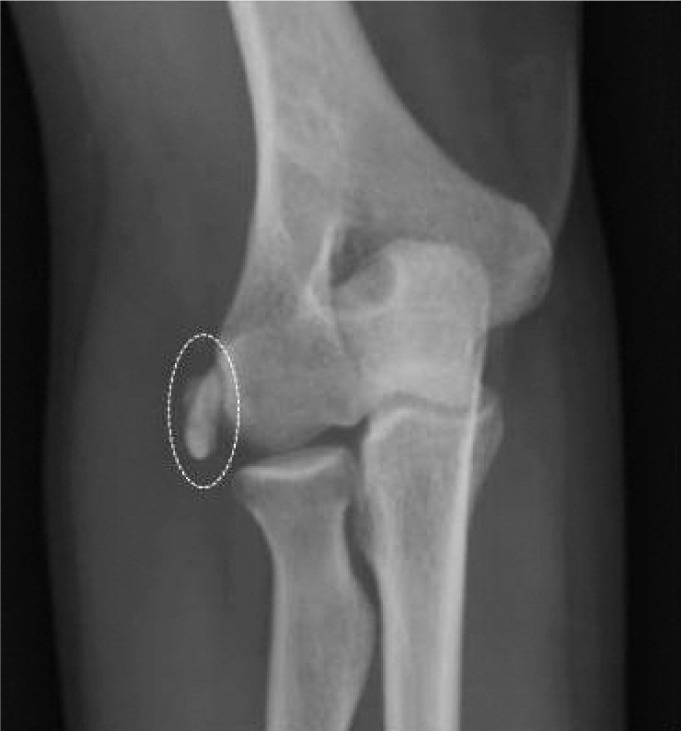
Anterior-posterior radiograph of right elbow, showing the presence of a large calcification in the soft tissues near the lateral epicondyle.
